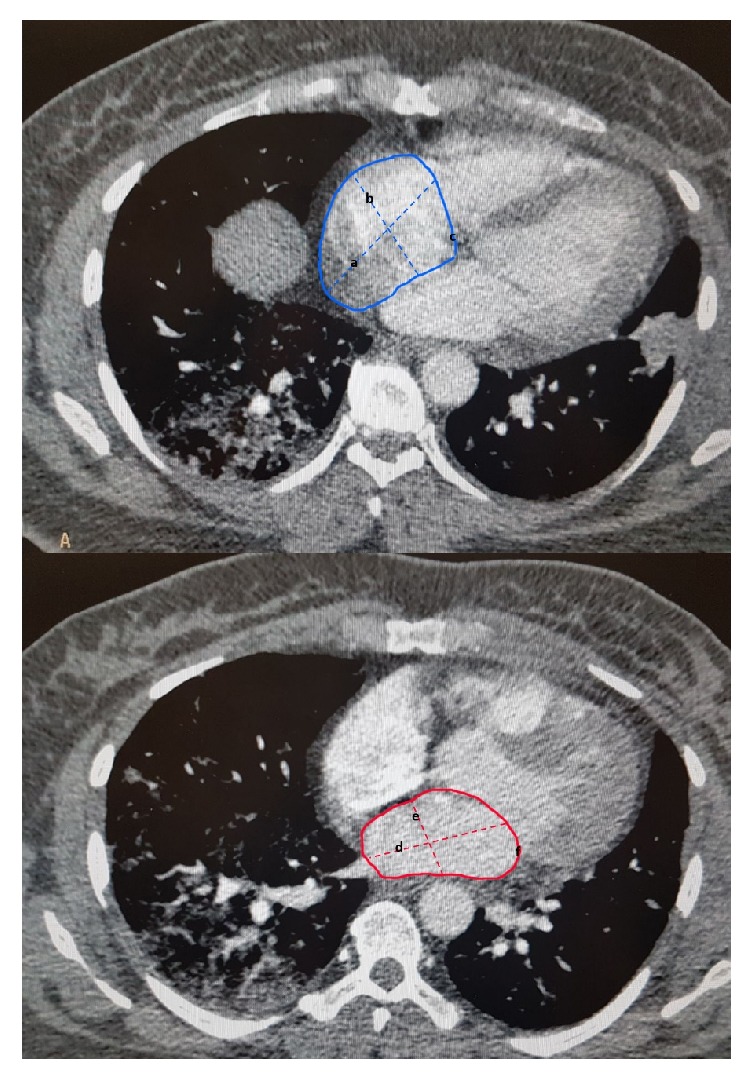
Radiological indices defined in computed tomography pulmonary angiography (CTPA). The figure, depicting a sample of computed tomography pulmonary angiography (CTPA) image and the indices defined for measuring different sizes. a, b, and c imply RA long diameter, short diameter, and area, respectively. d, e, and f imply LA long diameter, short diameter, and area, respectively.
